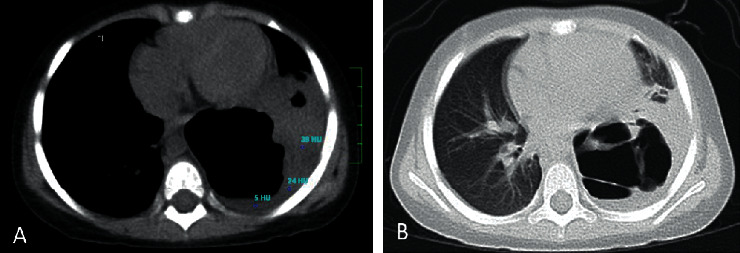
Noncontrast axial-chest CT: mediastinal window (a) and lung window (b). Left pleural effusion density was <25 HU. There was a poorly visible portion of the left lung, and there was air-filled thin wall cavity. Without IV contrast, cavitary necrosis could not be diagnosed because there was no enhancement of the surroundings.
